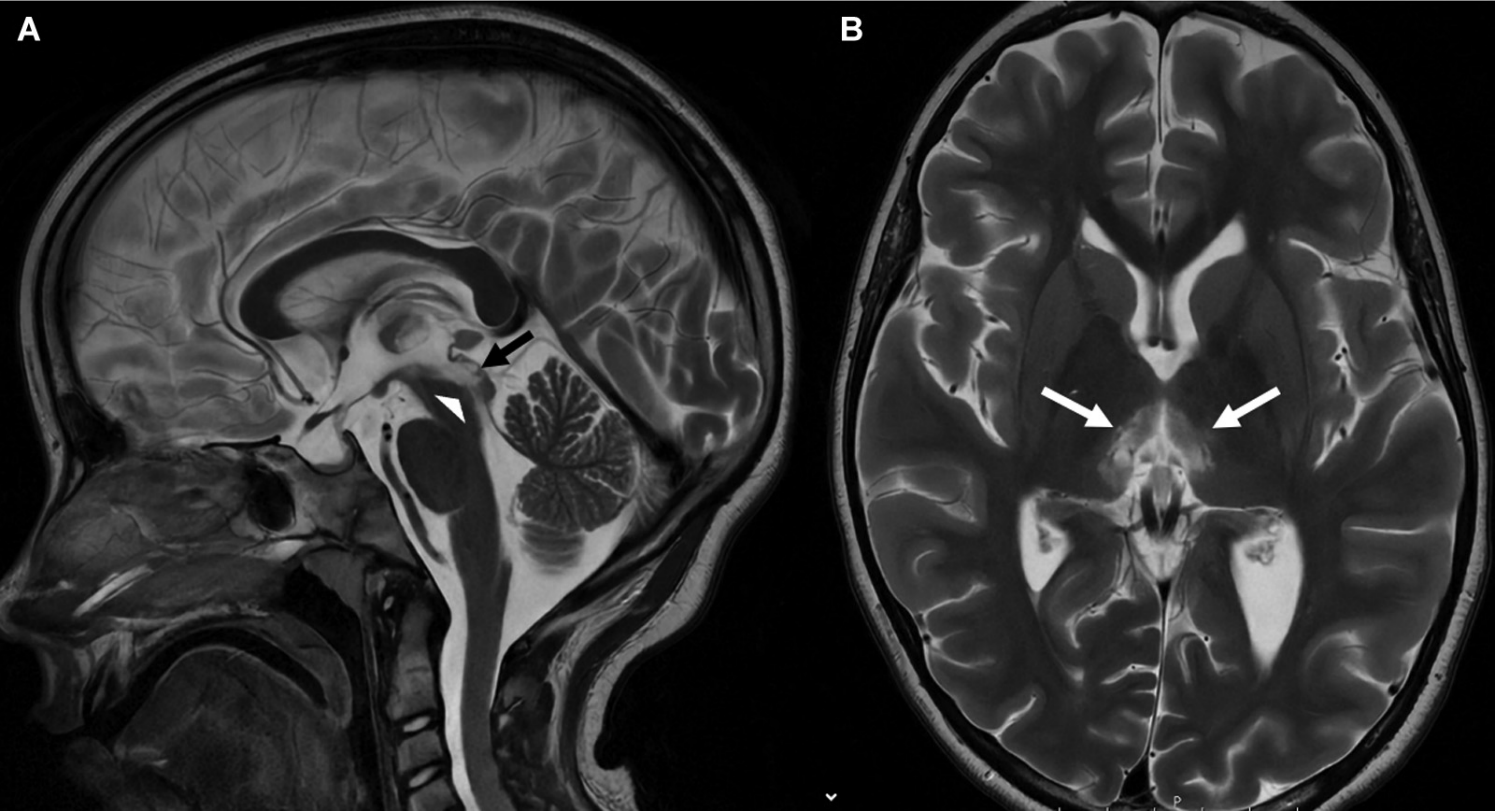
Follow-up magnetic resonance imaging after 3 weeks (T2-weighted sequences sagittal [
